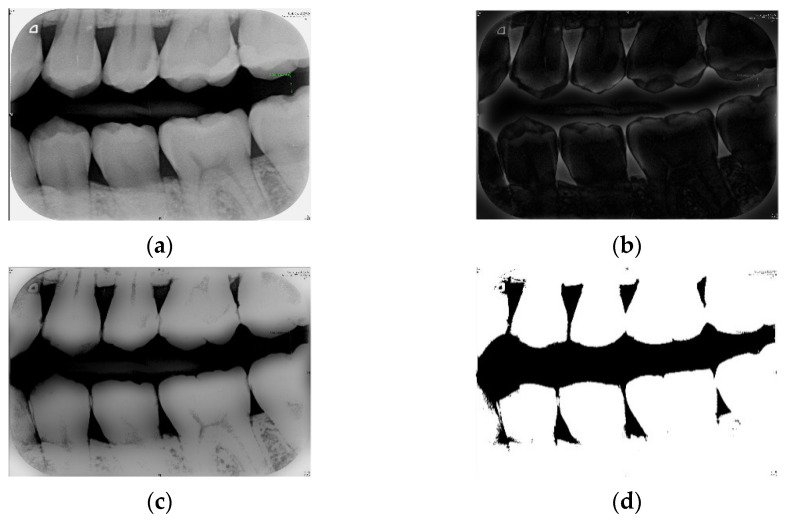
(a) The bitewing film; (b) the filtered result of (a) using the Gaussian high-pass filter; (c) the result of (a) minus (b); (d) the result of (c) after the binarization.
